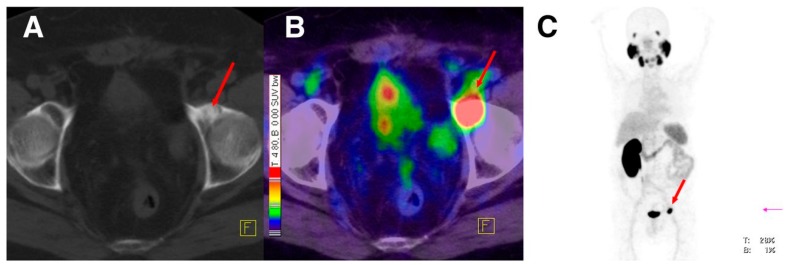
(A–C) ^68^Ga-PSMA-617 PET/CT of a patient at 1 h after injection. Red arrows point to a bone metastasis with SUVmax of 21.7 at 1 h and 32.6 at 3 h after injection. (A) Low dose CT; (B) Fusion of PET and CT; (C) MIP of PET/CT. MIP = maximum-intensity projection (Reprinted with permission of [146]).
